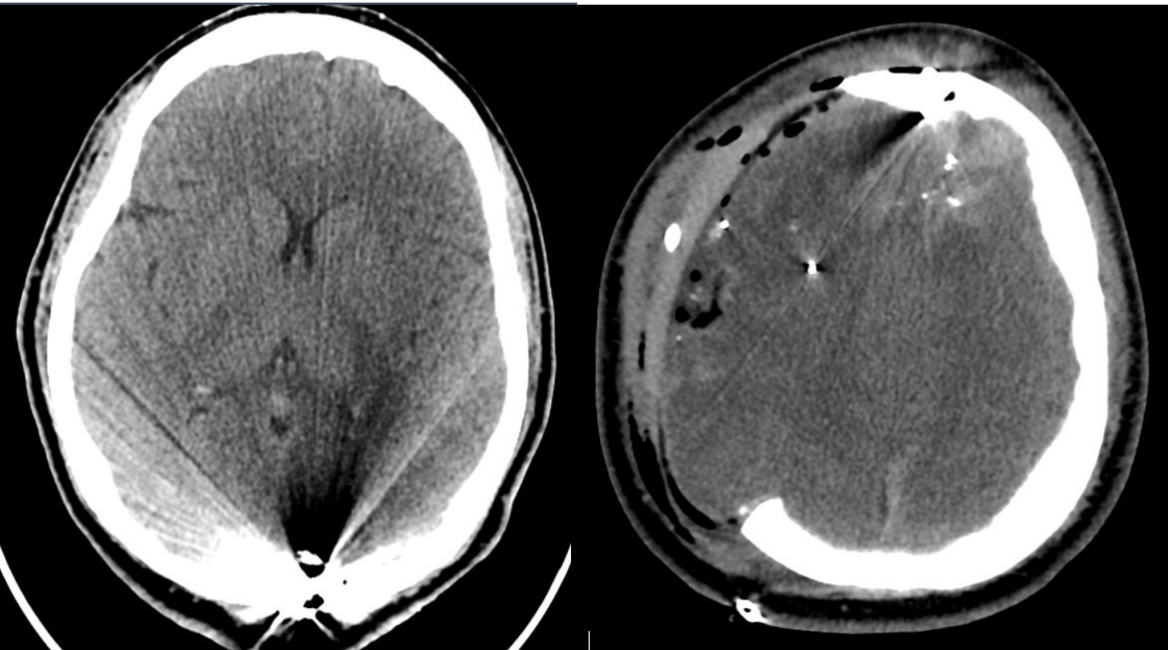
Post-operative computed tomography (CT) head revealed residual subdural hematoma, intraparenchymal hematoma, pneumocephalus, right greater than left edema, craniectomy defect herniation, and a small subgaleal hematoma.
